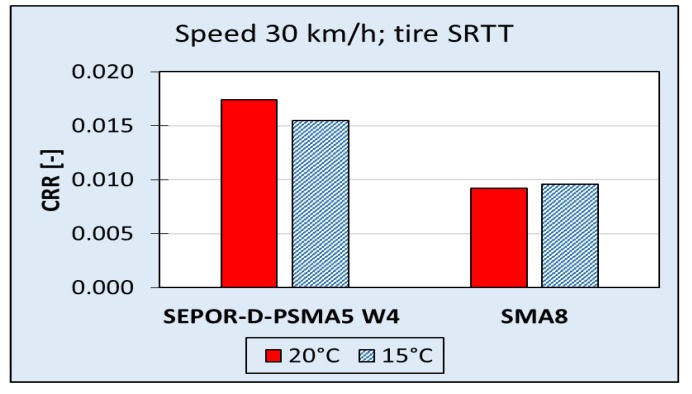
Coefficient of Rolling Resistance (CRR) for SEPOR-D-PSMA5 W4 and reference pavement SMA8 measured at the Dąbrówka section.
